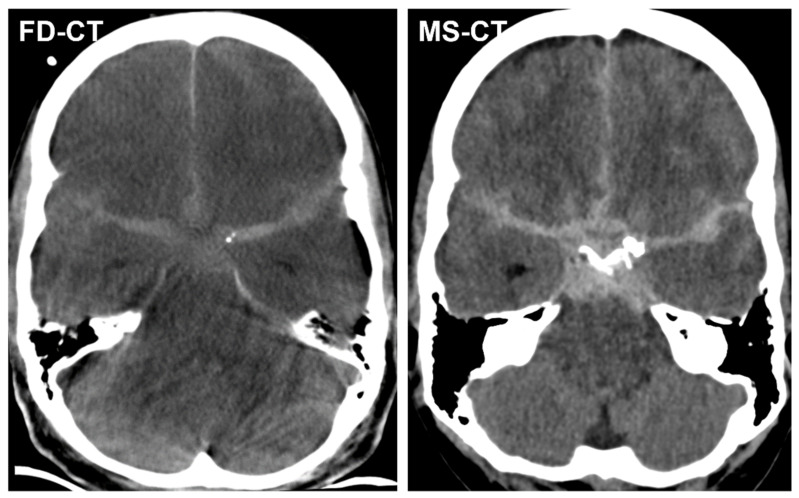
Exemplary flat-detector computed tomography (FD-CT) and multislice computed tomography (MS-CT) images of a patient with an endovascular treated, aneurysmal subarachnoid haemorrhage (SAH). Both FD-CT and MS-CT allow the delineation of the SAH as hyperdense filling of the subarachnoid spaces around the circle of Willis.
